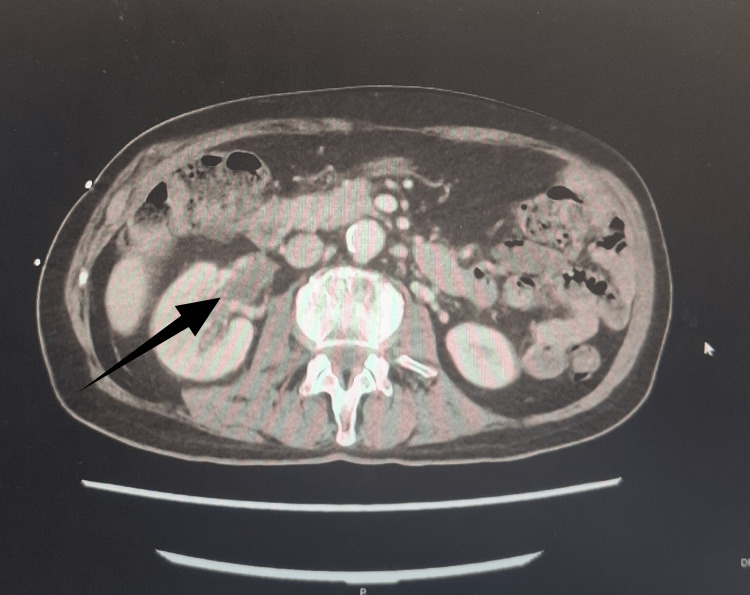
Contrast-enhanced CT scan of the abdomen in the axial view showing features suggestive of pyelonephritis. The black arrow points to the pathology on the CT scan.
